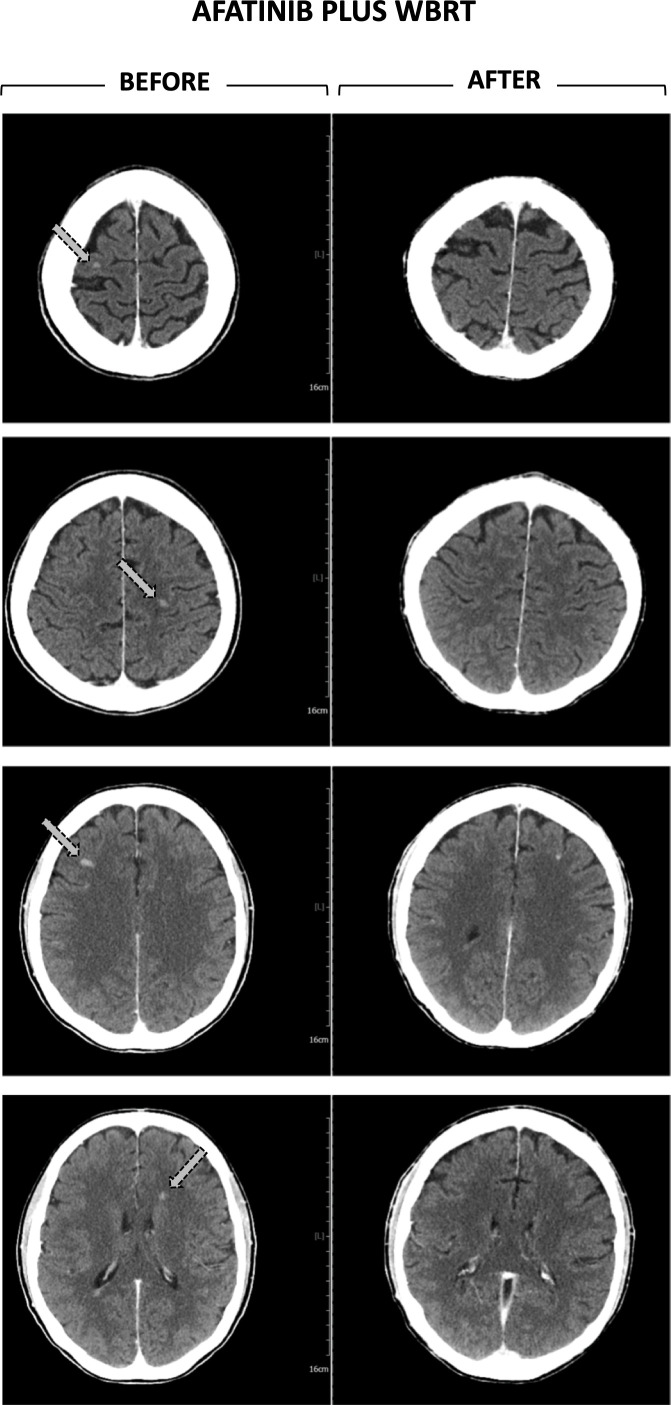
CT scan. Left Panel. Total body CT performed 10 months after the first diagnosis of NSCLC, showing for the first time, BMs. Right Panel. Total body CT performed two month and a half after the end of the WBRT, showing stable BMs.
